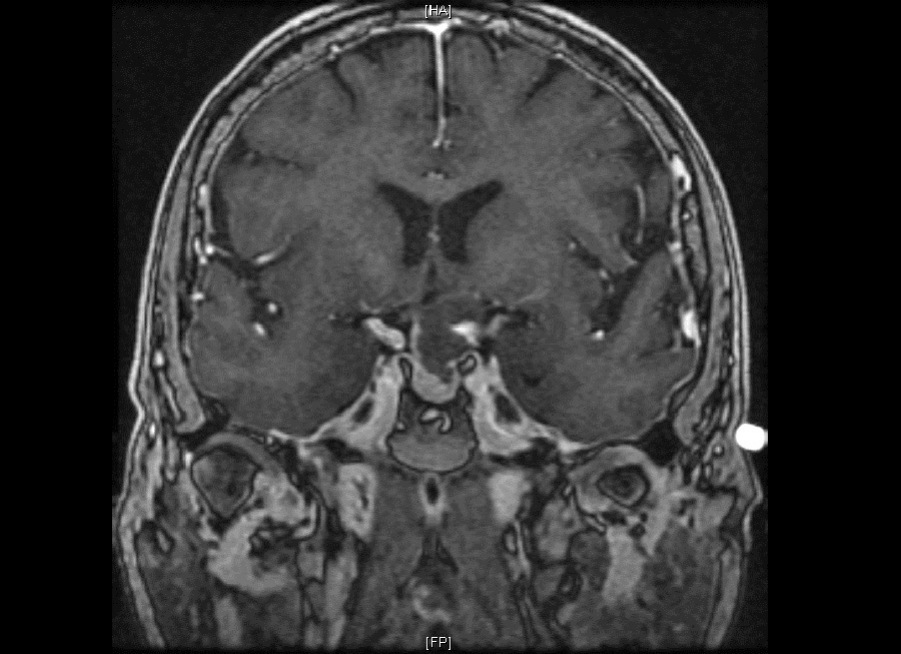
Preoperative coronal T1 MRI with contrast shows a suprasellar solid-cystic mass compressing the optic chiasm
